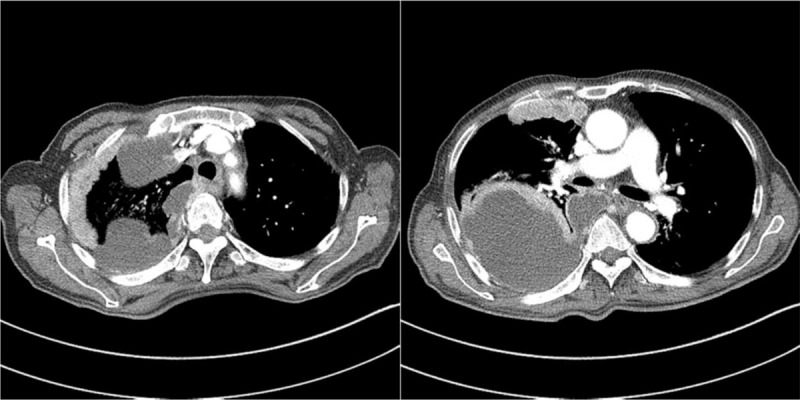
Chest computed tomography revealed massive right pleural effusion and diffuse nodular thickening with passive atelectasis in the right middle lung and right lower lung fields.
